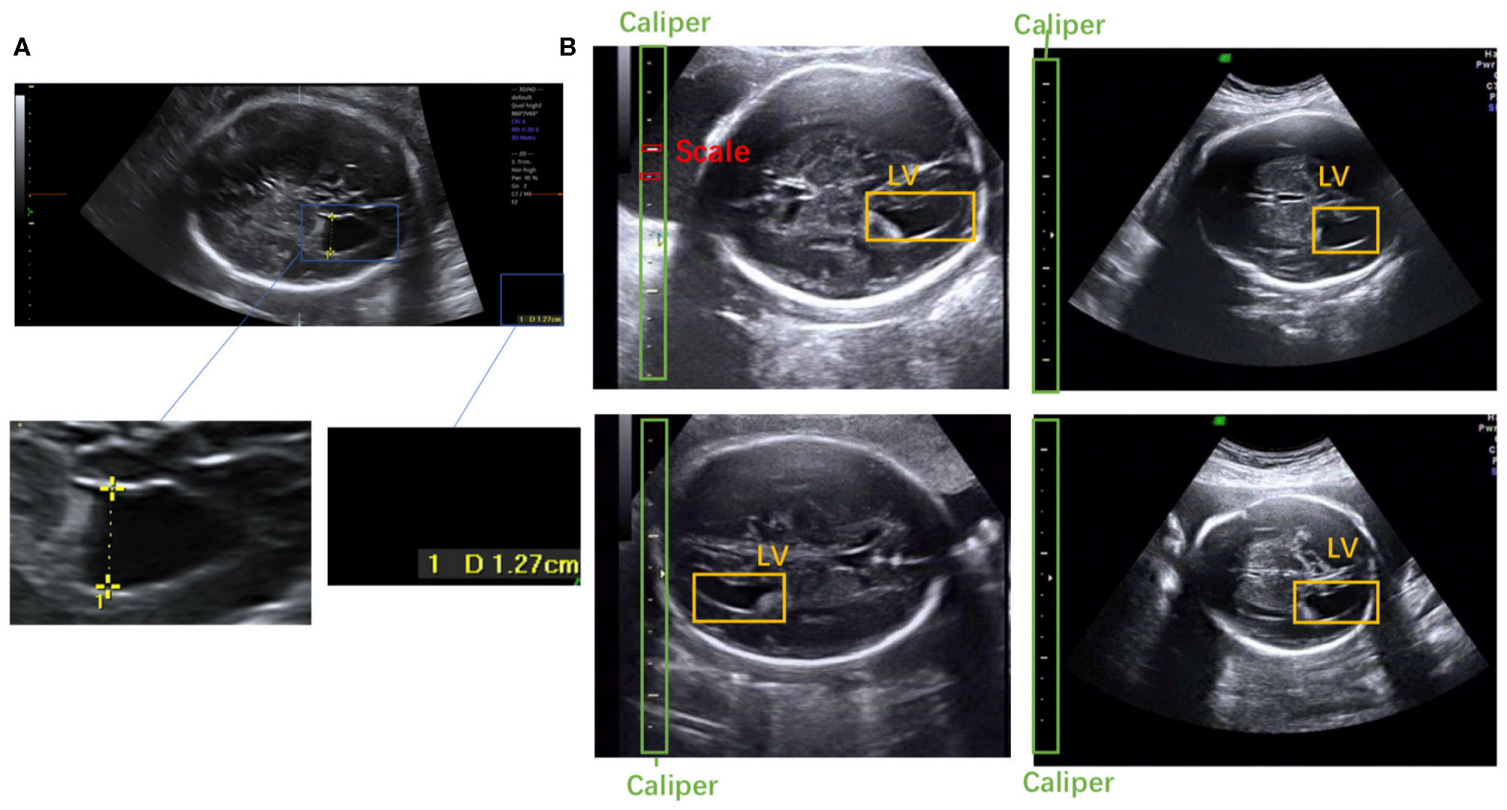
(A) Scanner-located LV width; the yellow crosses represent the endpoints, and the physical length of the LV is shown in the lower right corner. (B) Fetal LV images in different ultrasound instruments.
